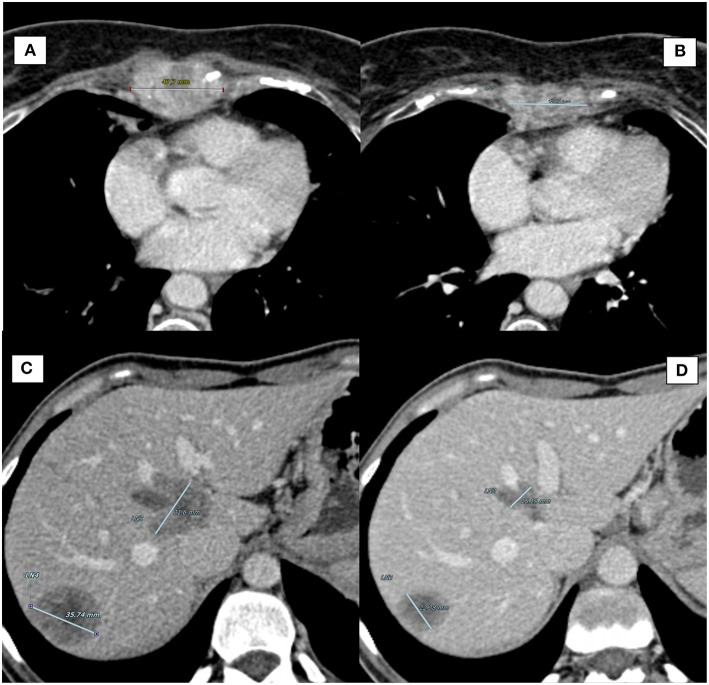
Computed Tomography (CT) scan of target lesions during therapy with CDK4/6 inhibitors. (A) Case report 1, sternal lesion at baseline (longest diameter: 47.7 mm). (B) Case report 1, sternal lesion at best response (longest diameter: 42.91 mm). (C) Case report 2, largest liver metastasis at baseline (longest diameter: 35.74 mm). (D) Case report 2, largest liver metastasis at best response (longest diameter: 22.38 mm).
